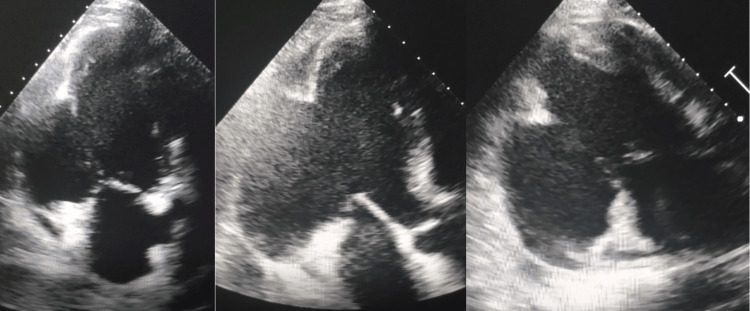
TTE showing a large submitral aneurysm free of thrombus with a wide neck. TTE: transthoracic echocardiography.
